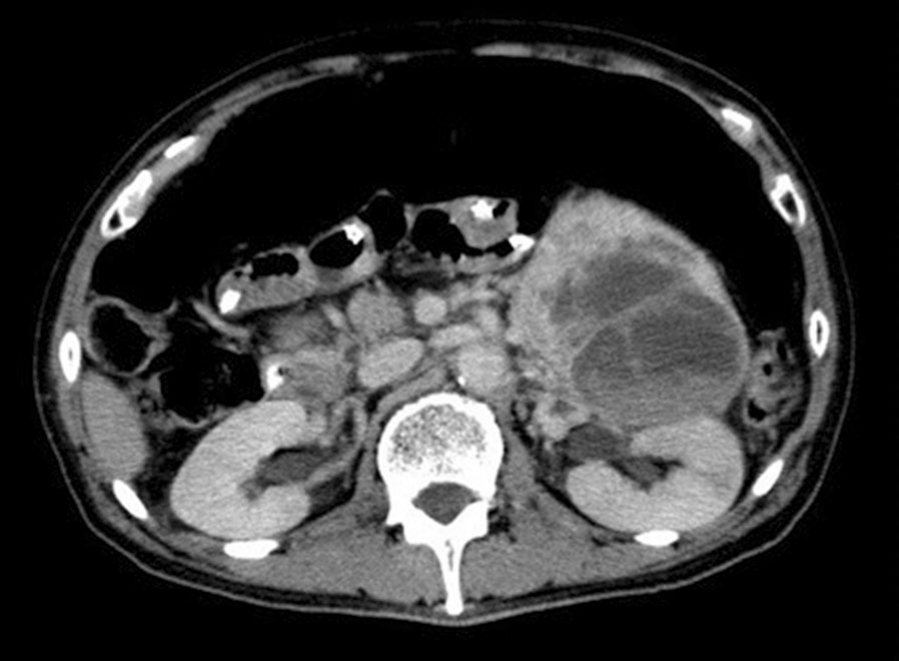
Abdominal computed tomography revealed a large left adrenal mass with heterogeneous enhancement and extended intensity compatible with ileus
